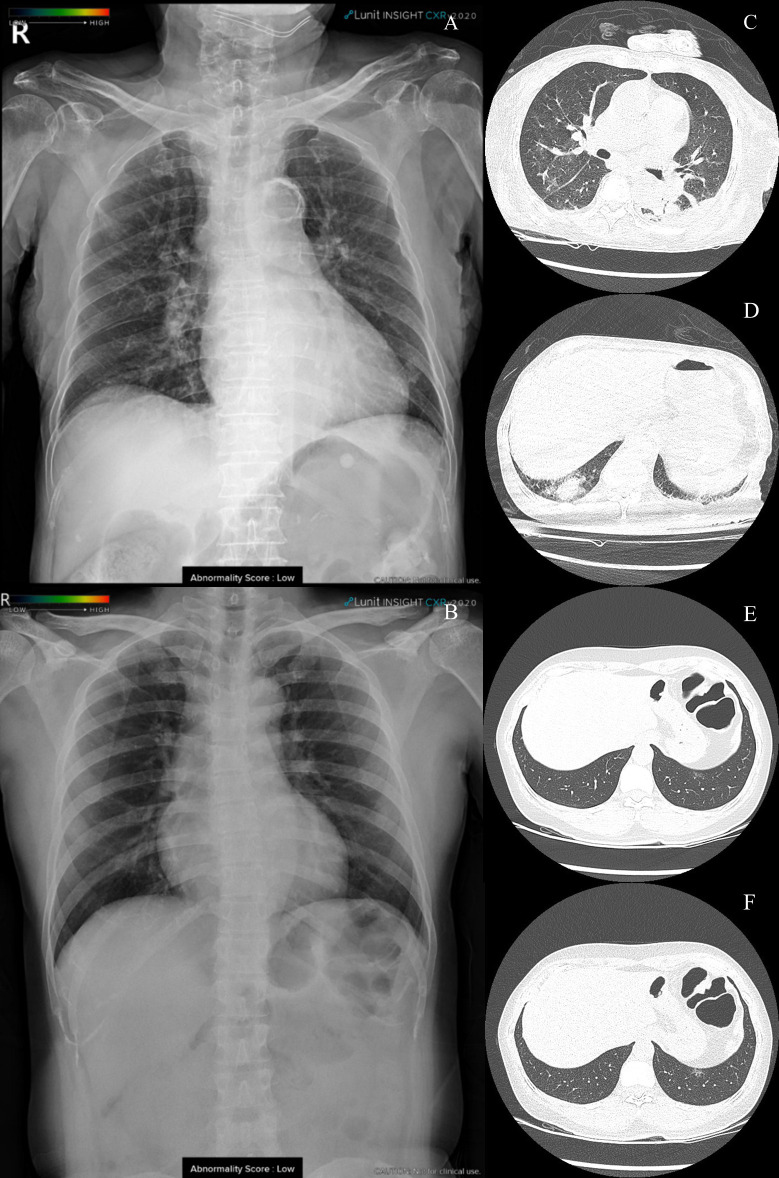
False-negative interpretations of DL algorithm. (A, B) DL algorithm classified chest radiography as negative for pneumonia. Chest computed tomography scans show (C) focal GGOs in the right middle lobe, (D) multifocal consolidations in the right lower lobe, and (E, F) small amounts of GGOs.
